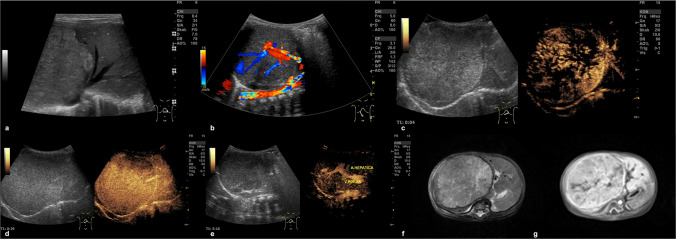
Hepatoblastoma in a neonate born at 41 ^1^/_7_ weeks’ gestation, presented on the ninth day of life (weight 3.46 kg, length 52 cm). Tumor in the whole right liver lobe (segments V–VIII), measuring 9.2 × 8 × 9 cm (~ 330 mL). AFP markedly elevated at 1.370574 µg/L (normal < 41.687 µg/L). The patient received three cycles of cisplatin-based chemotherapy followed by right hemihepatectomy, resulting in sustained remission. a Subcostal view. B-Mode image through the right and left liver lobe showing an echorich, well-delineated tumor within the whole right liver lobe obstructing the right liver vein. No portal venous branches can be seen within the tumor. The parenchyma of the left liver lobe is normal. b Doppler scan in an oblique-longitudinal section through the liver vessels and the aorta. Marked increase of the diameter and hepatic artery flow due to tumoral needs. c In the early arterial phase (4 s post-injection), CEUS shows multiple tortuous tumor vessels appearing at the same time in the periphery and the center of the tumor. This underlines again the high diagnostic value of the early arterial phase. d In the beginning of the portal venous phase of CEUS the hepatoblastoma is now completely enhanced. e CEUS 5 min and 16 s post-injection, subcostal oblique view. Marked washout within the hepatoblastoma in the right liver lobe, but still good enhancement in the portal vein and the hepatic artery. No signs of vessel infiltration or tumor thrombosis. f Axial T2-weighted MRI with fat suppression showing a liver tumor with heterogeneous structure and minimal central necrosis. g Axial post-contrast T1-weighted MRI with fat suppression demonstrating marked contrast enhancement of the liver tumor
